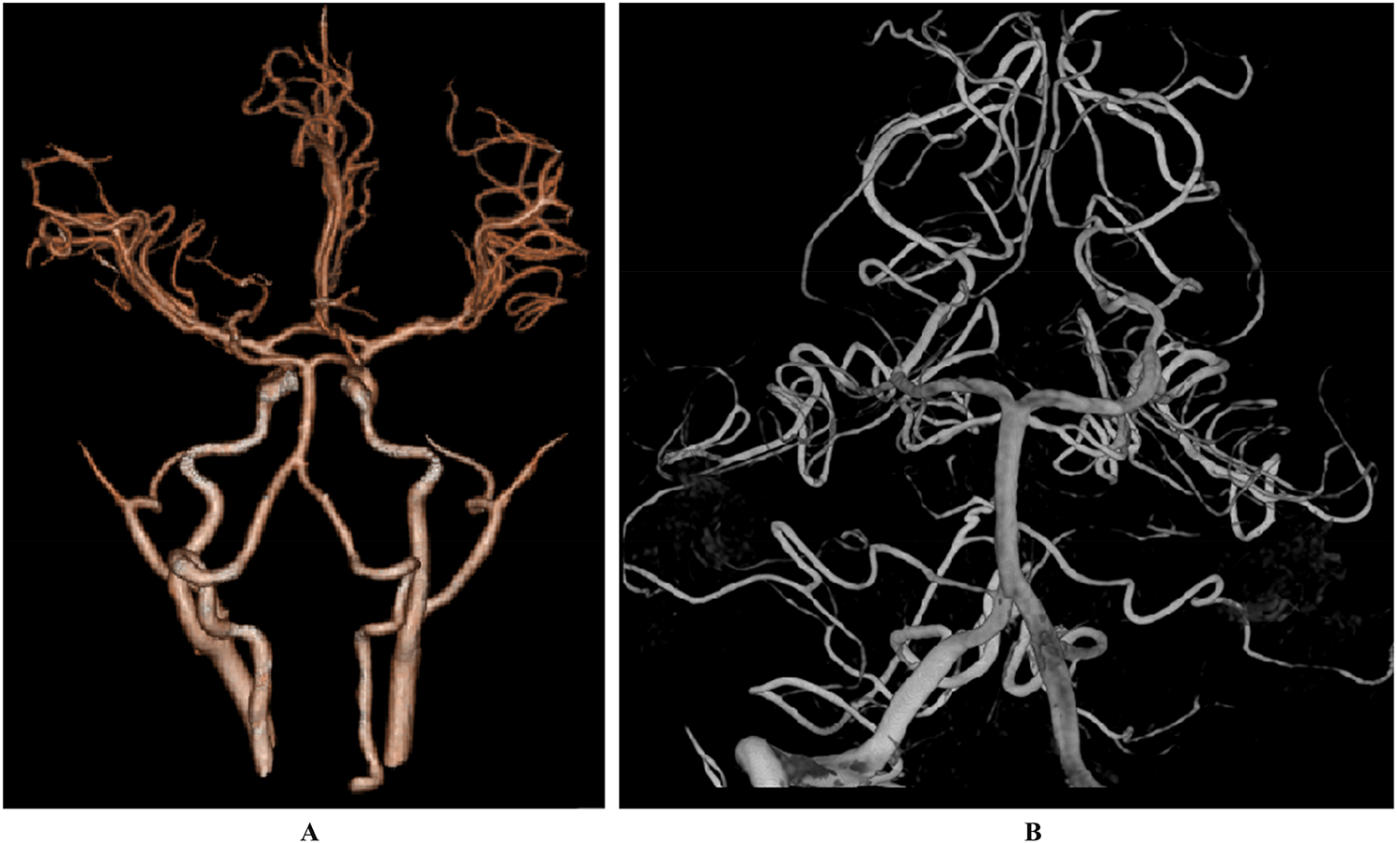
The results of CTA (A) and DSA (B) did not show any significant abnormalities.
